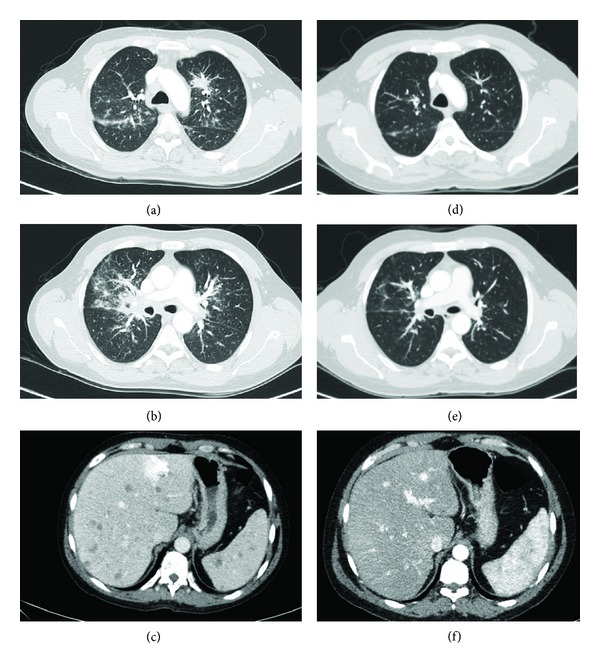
Chest ((a), (b), (d), and (e)) and abdominal ((c), (f)) CT scans showing resolution after treatment ((d), (e), and (f)) of diffuse lesions ((a), (b), and (c)).
